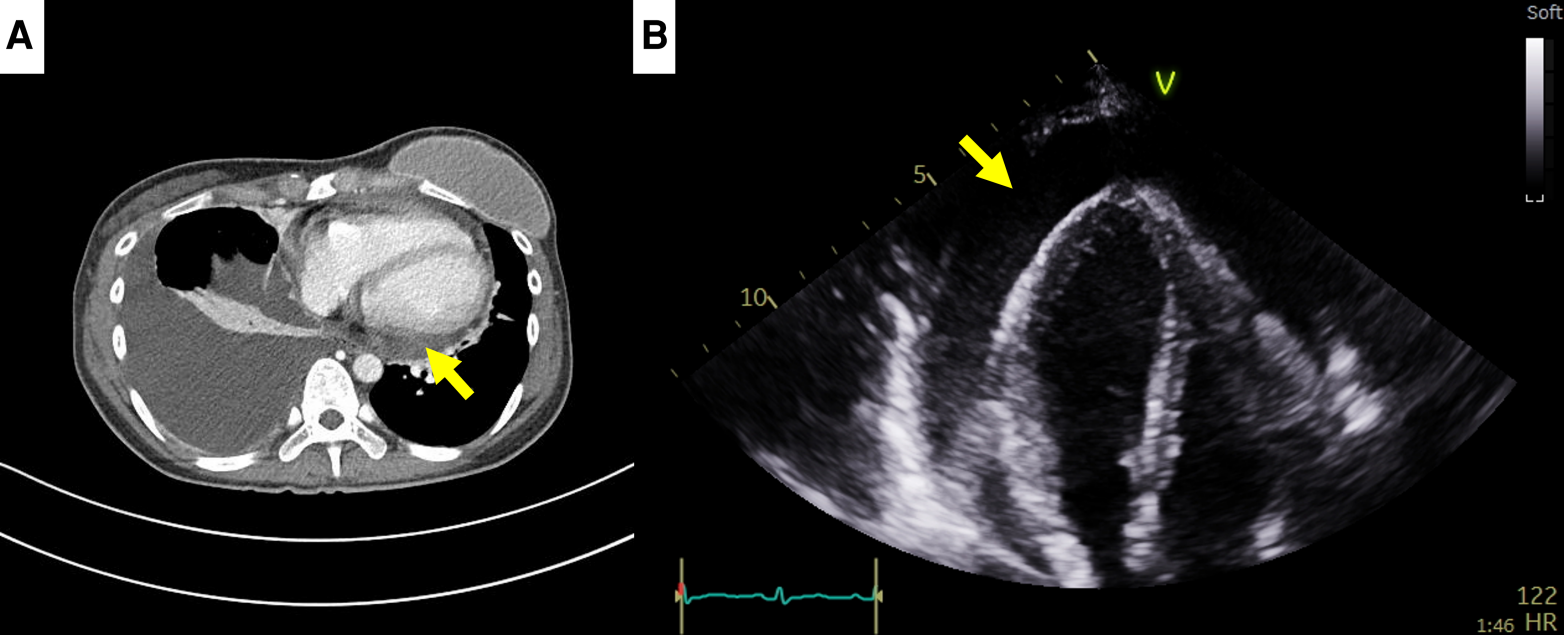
Computed tomography (CT) findings of case 2. (A) Small pericardial effusion in chest CT, 1 month prior to cardiac tamponade episode. (B) Large pericardial effusion (arrow) causing cardiac tamponade, 1 month after chest CT.
